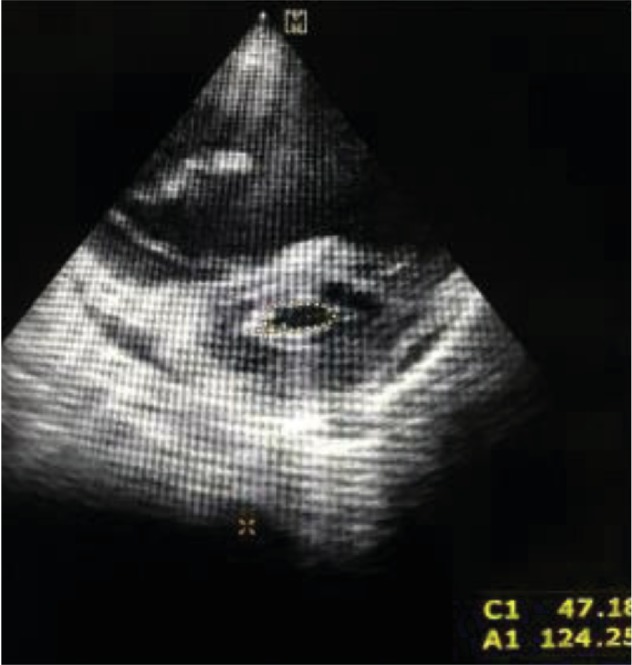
2D echocardiogram-guided planimetry showing MVA is 1.24 cm^2^.
